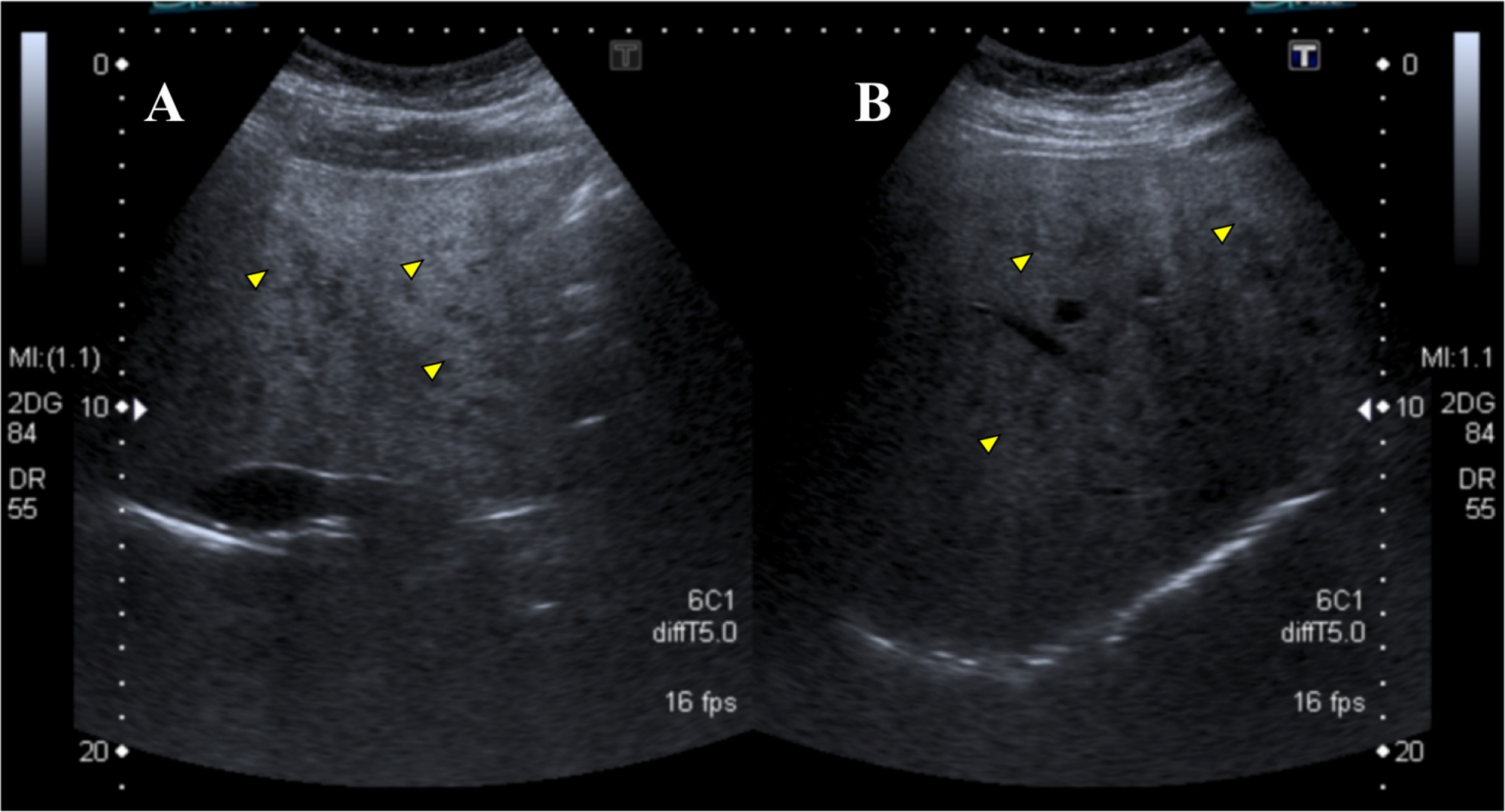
Abdominal ultrasound: diffuse hepatic micronodular involvement (arrowheads) (A) Epigastric transverse scanning. (B) Right subcostal scanning
